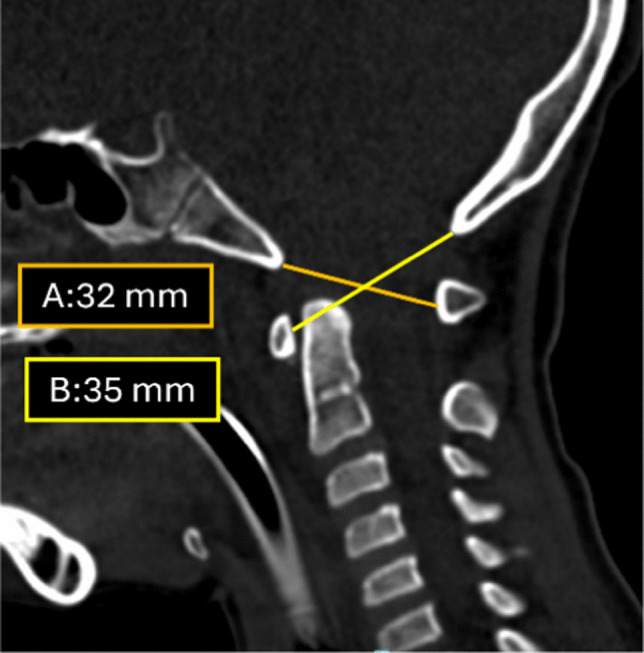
Sagittal CT in a 6-year-old with cervical trauma. Distance *A* is measured from the inferior tip of the basion to the anterior mid cortex of the posterior arch of C1 and distance *B *is measured from the inferior tip of the opisthion to the posterior mid cortex of the anterior arch of C1. Ratio of distances *A* and *B* gives the Power ratio [32/35] which is <1 (normal)
